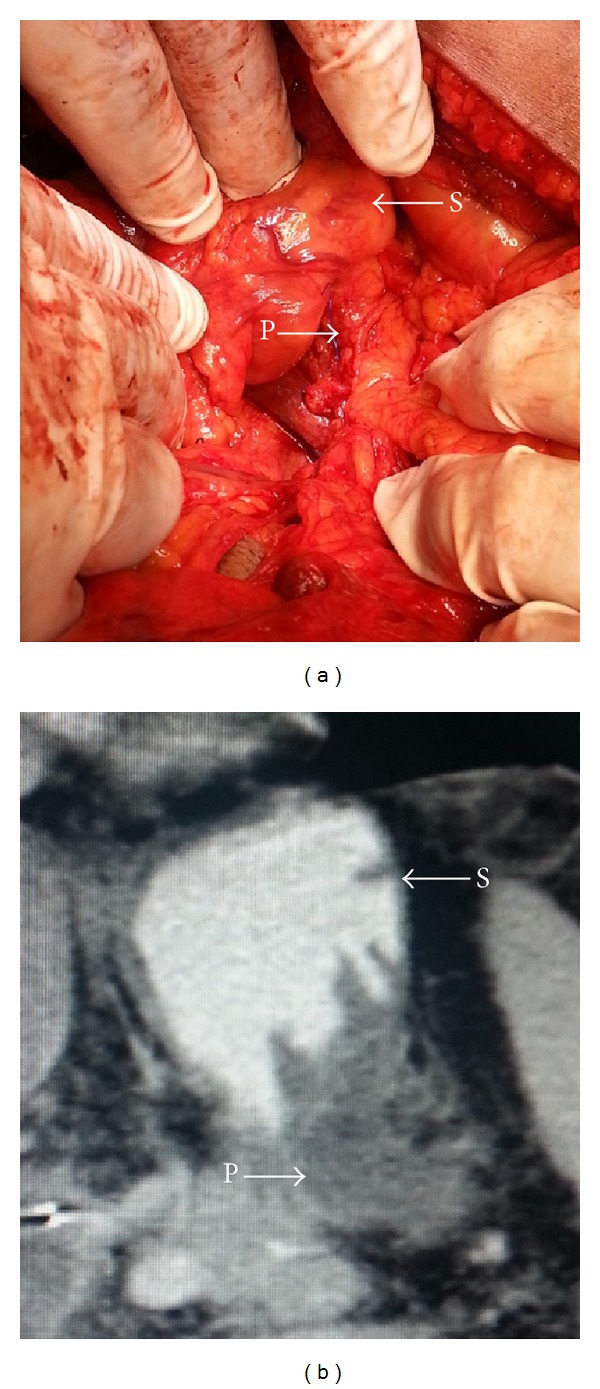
(a) Intraoperative invagination of the pancreatic remnant (P) into the posterior gastric wall (S); (b) postoperative CT scan showing invagination of the pancreatic remnant (P) into the posterior gastric wall (S).
